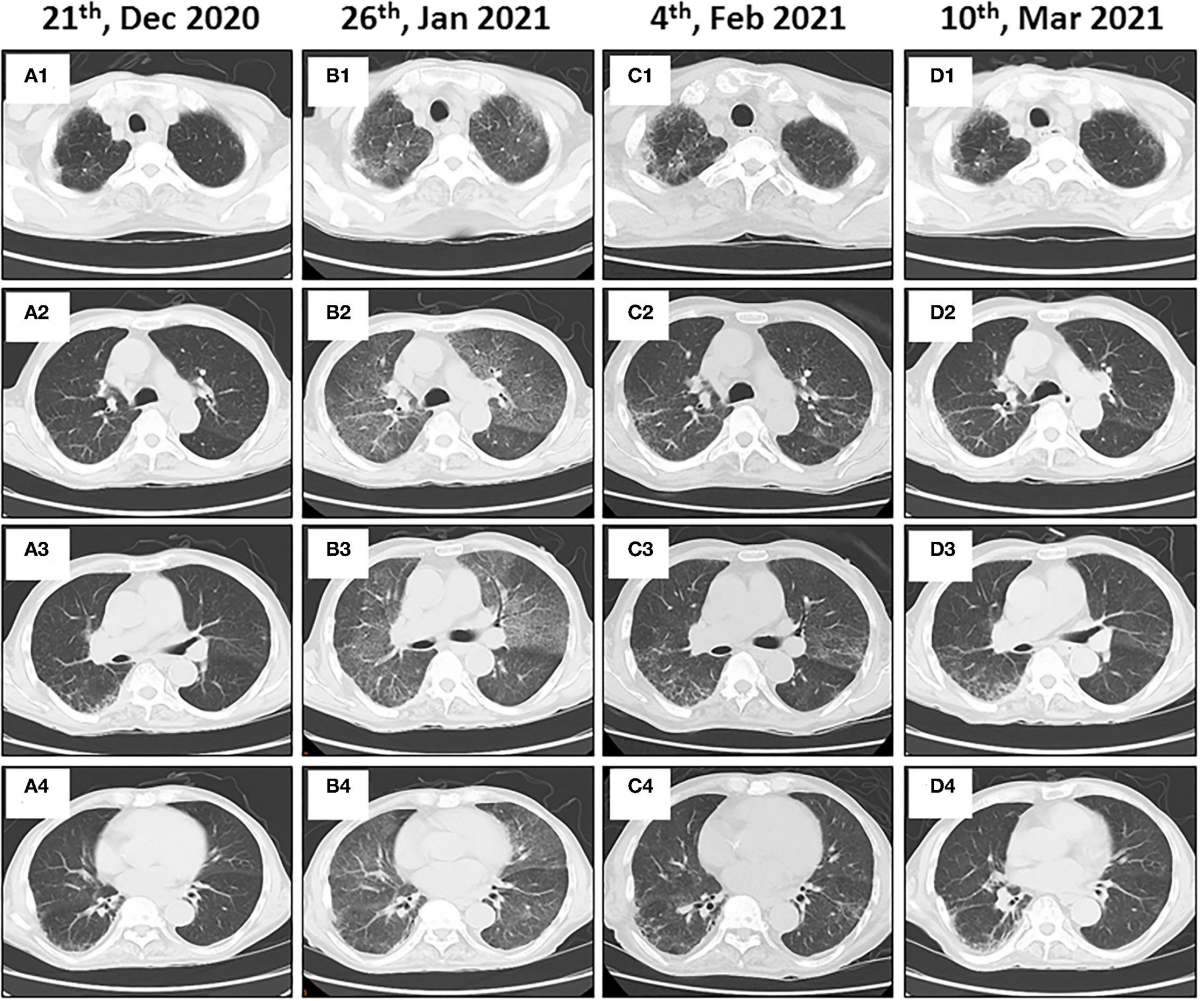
Immune-related pneumonitis in a patient with metastatic gastric cancer and rare severe immune-related cholestatic hepatitis and subsequent severe late-onset pneumonia after pembrolizumab therapy. Baseline chest computed tomography (CT) images show the lungs before starting immunotherapy (A1–A4). Follow-up axial CT images obtained 4 months after administering the pembrolizumab therapy showing non-specific interstitial pneumonia (B1–B4). Immunotherapy was halted, and steroid therapy was administered. Follow-up chest CT images show near complete resolution of pneumonitis (C1–C4). Chest CT images obtained after the steroid therapy was discontinued for 5 weeks (D1–D4).
